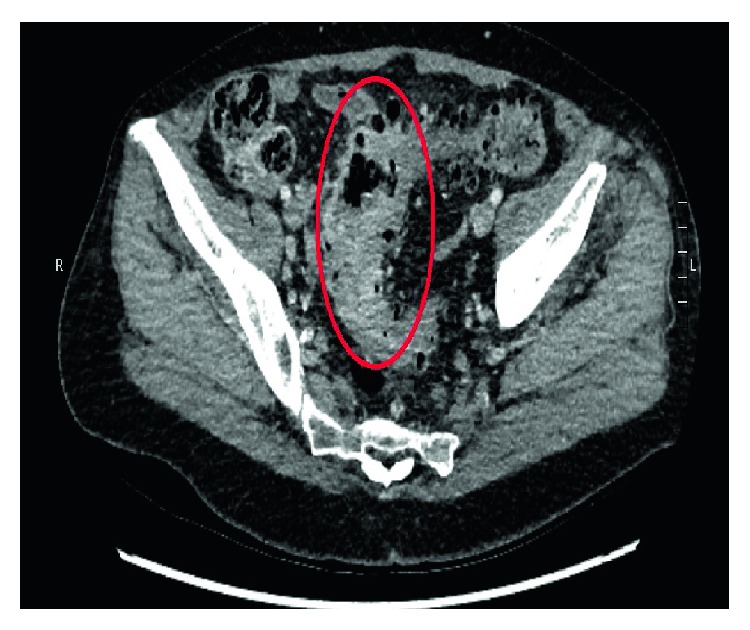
Axial CT highlighting a focal area of thickening in the wall of the sigmoid colon with surrounding diverticula.
